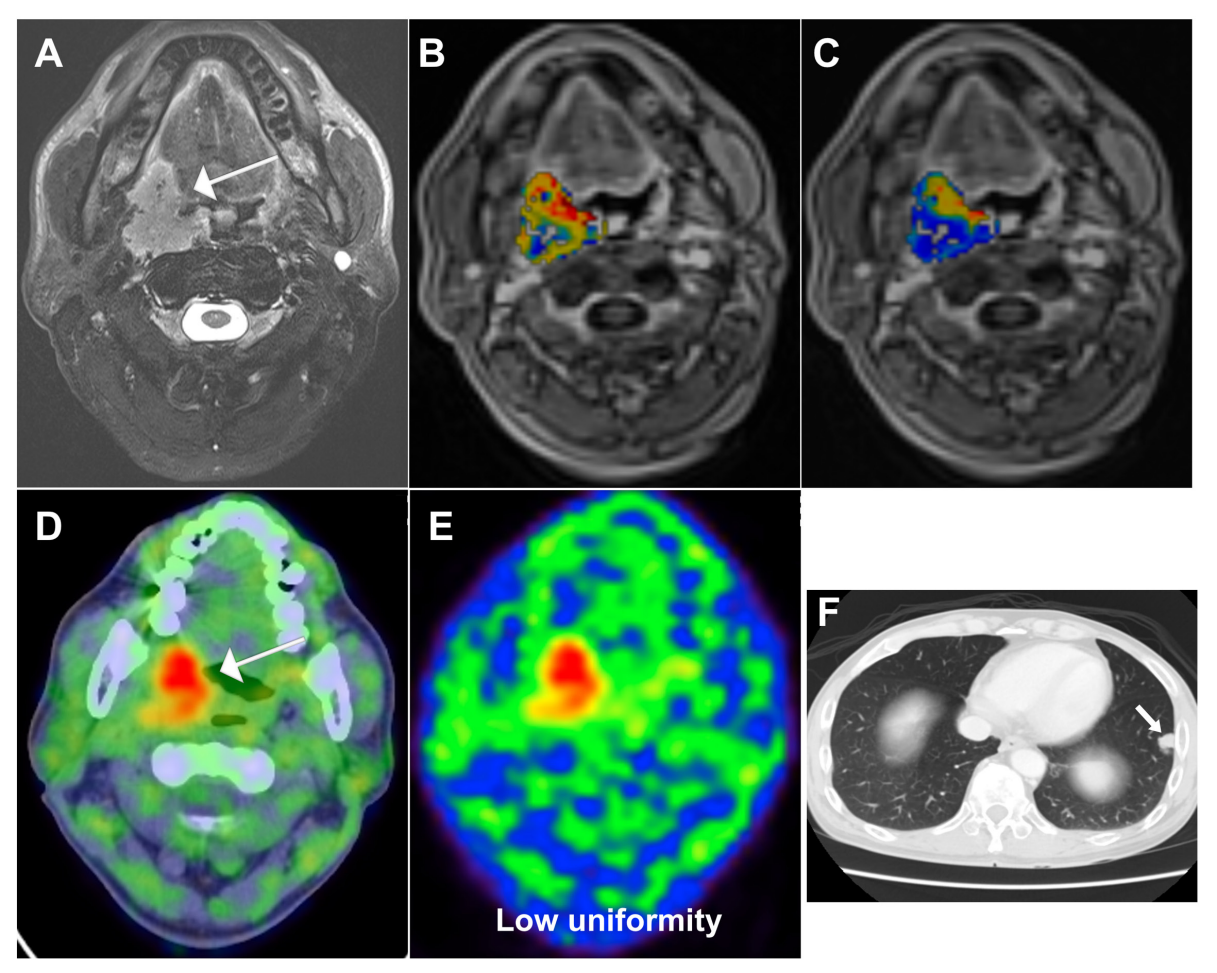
A 51-year-old male patient with oropharyngeal squamous cell carcinoma and a score of 4 based on the prognostic system developed in the current study A. Pretreatment axial-enhanced MRI image showing a right oropharyngeal tumor (arrow). B. The corresponding DCE-MRI image with an overlaid K^*trans*^ map of the primary tumour showed a value of 0.5116 min^−1^. C. The corresponding DCE-MRI image with an overlaid K_*ep*_ map of the primary tumor showed a value of 1.3648 min^−1^. D., E. The corresponding ^18^F-FDG PET/CT image showed a uniformity value of 0.0032 and a SUV of 8.92 g/mL. F. The patient developed a distant recurrence to the lung and died 10 months thereafter. Of note, this case would have been classified as having a favorable outcome (with a score of 1) using the modified prognostic scoring system reported in the previous study (Table 3).
